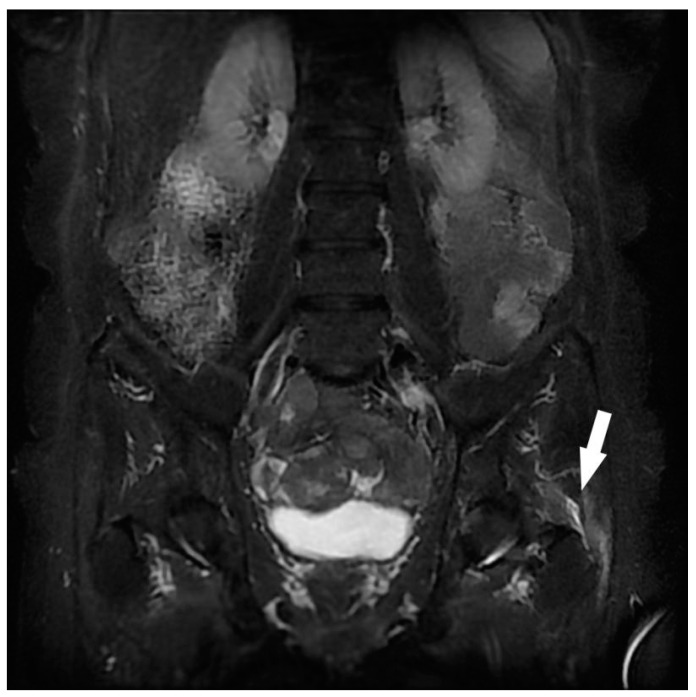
Assessment of left sciatica in a 72-year-old woman. Coronal STIR MRI. Hypersignal at the height of the left peri-trochanteric region, of the small and medium gluteal tendons with effusion within their purse. Left gluteal tendinobursitis (white arrow).
